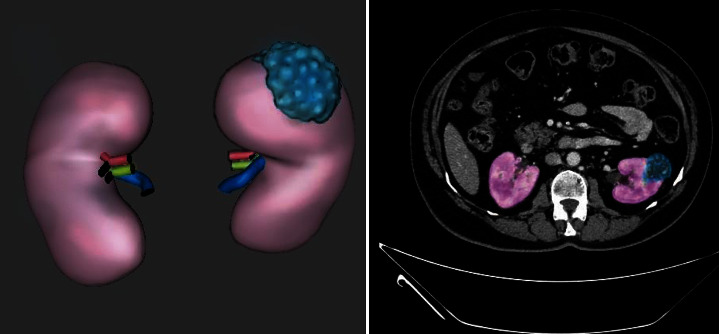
Sample renal CT taken from the dataset.
